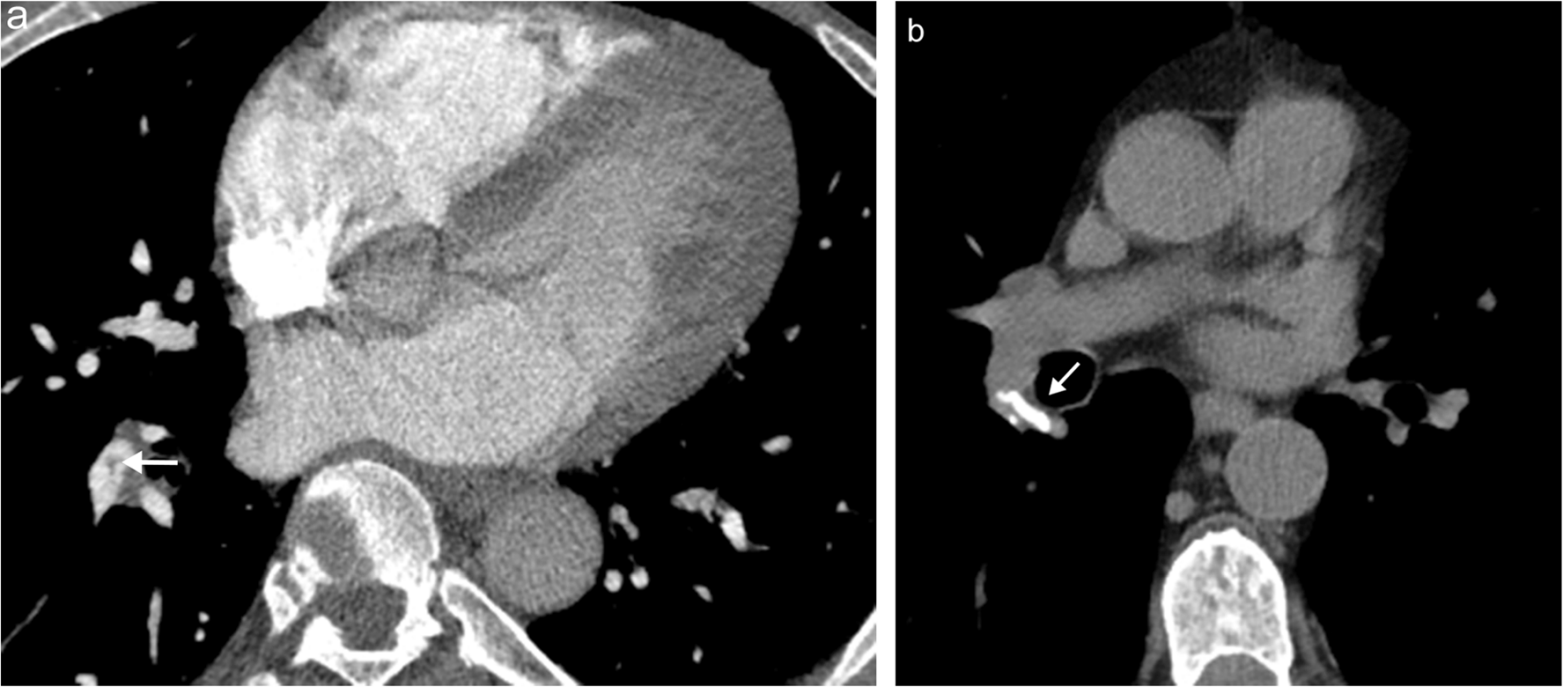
Two patients with chronic pulmonary emboli. a Axial contrast-enhanced CT image shows a web-like filling defect within a segmental right lower lobe pulmonary artery consistent with chronic pulmonary embolus (*white arrow*). b Axial non-contrast CT image of another patient demonstrates linear calcification within the right interlobar pulmonary artery consistent with chronic pulmonary embolus (*white arrow*)
